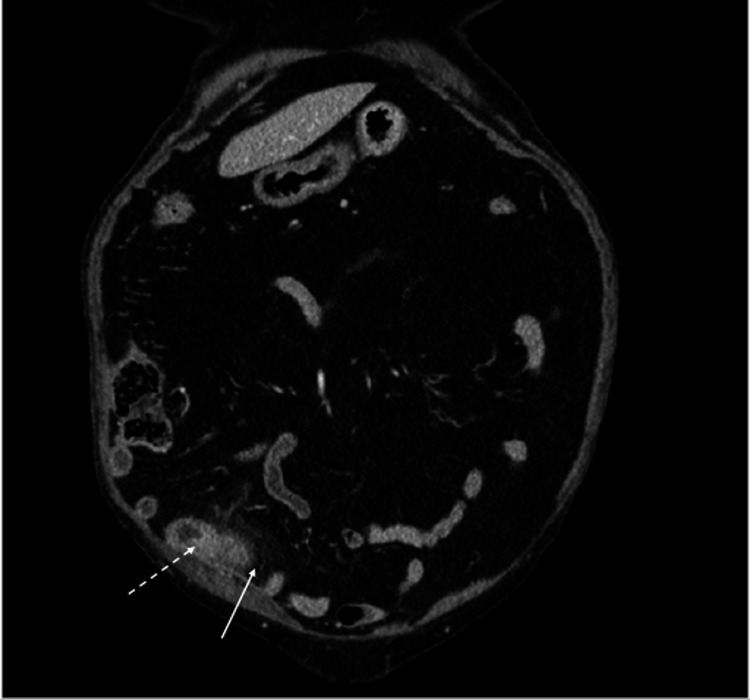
Coronal plane of CT scan of abdomen and pelvis with IV contrast showing the appearance of an abnormally distended appendix (dotted white arrow) and abnormal fat stranding in adjacent soft tissues (white arrow). CT: Computed tomography; IV: Intravenous
